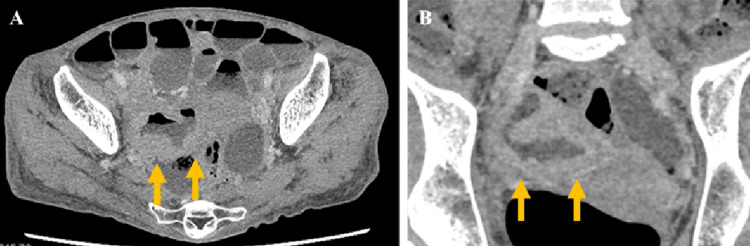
Abdominal computed tomography (CT) images on initial examination Transverse (A) and coronal (B) CT images show thickening of the wall of the ileum (arrow).
